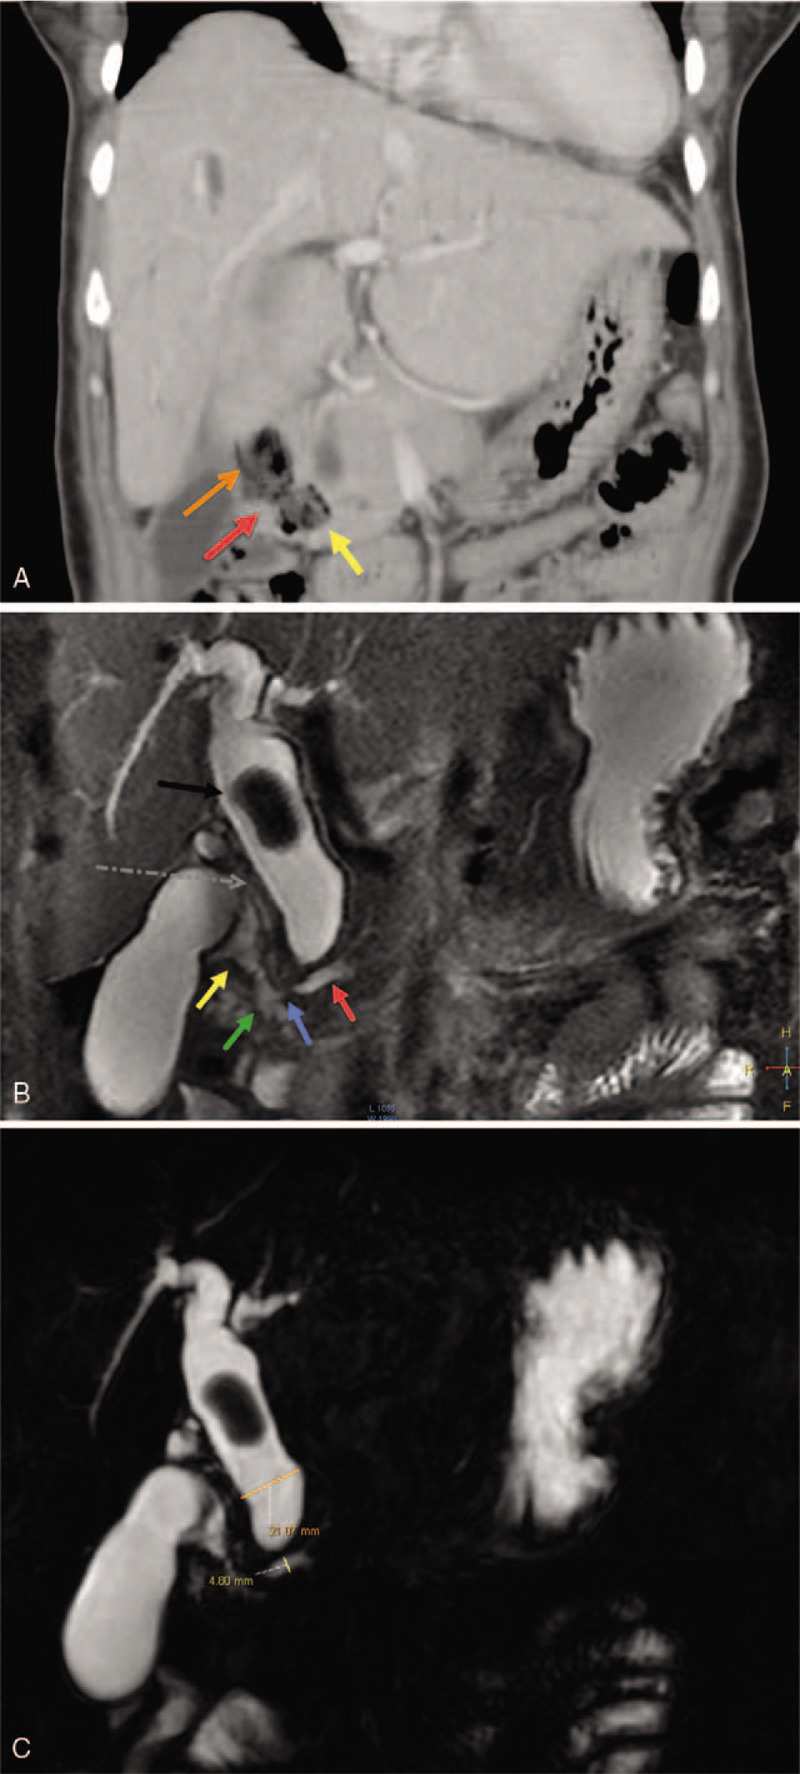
(A) An enhanced abdominal computed tomography scan showing coronal slice image obtained through the diverticulum (orange arrow). The narrow ostium (red arrow) connecting the diverticulum to the second part of the duodenum D2 (yellow arrow) is shown. (B) A magnetic resonance cholangiopancreatography image through the diverticulum showing coronal slice obtained through the diverticulum (yellow arrow). The 21 mm dilated common hepatic duct (black arrow) and an 18 mm floating choledocholith, a dilated omega-shaped cystic duct descending parallel to the common hepatic duct with low insertion to the common bile duct (dotted gray arrow), and a 4.8 mm dilated pancreatic duct of Wirsung (red arrow) with downstream stenosis (blue arrow) is shown, just before draining into the major papilla in the second part of the duodenum D2 (green arrow). (C) A magnetic resonance cholangiopancreatography scan of the dilated common hepatic artery showing a thick coronal slice image. A 21 mm dilated common hepatic duct (orange line), an 18 mm floating choledocholith, and a 4.8 mm dilated pancreatic duct of Wirsung (yellow line) are shown.
